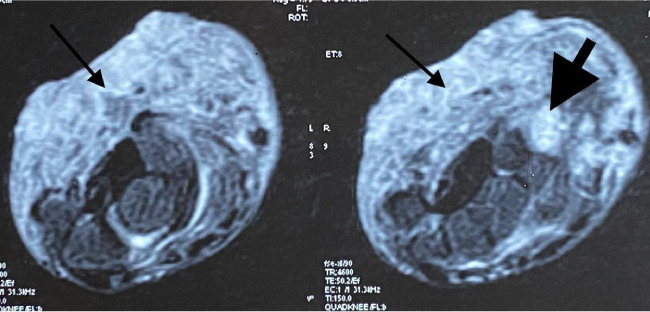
Magnetic resonance imaging images of the right wrist (short tau inversion recovery (STIR) axial) showing an ill-defined soft tissue lesion involving the flexor aspect of the distal third of the forearm and wrist (thin black arrow). Lesion involving the pisiform bone (thick black arrow).
